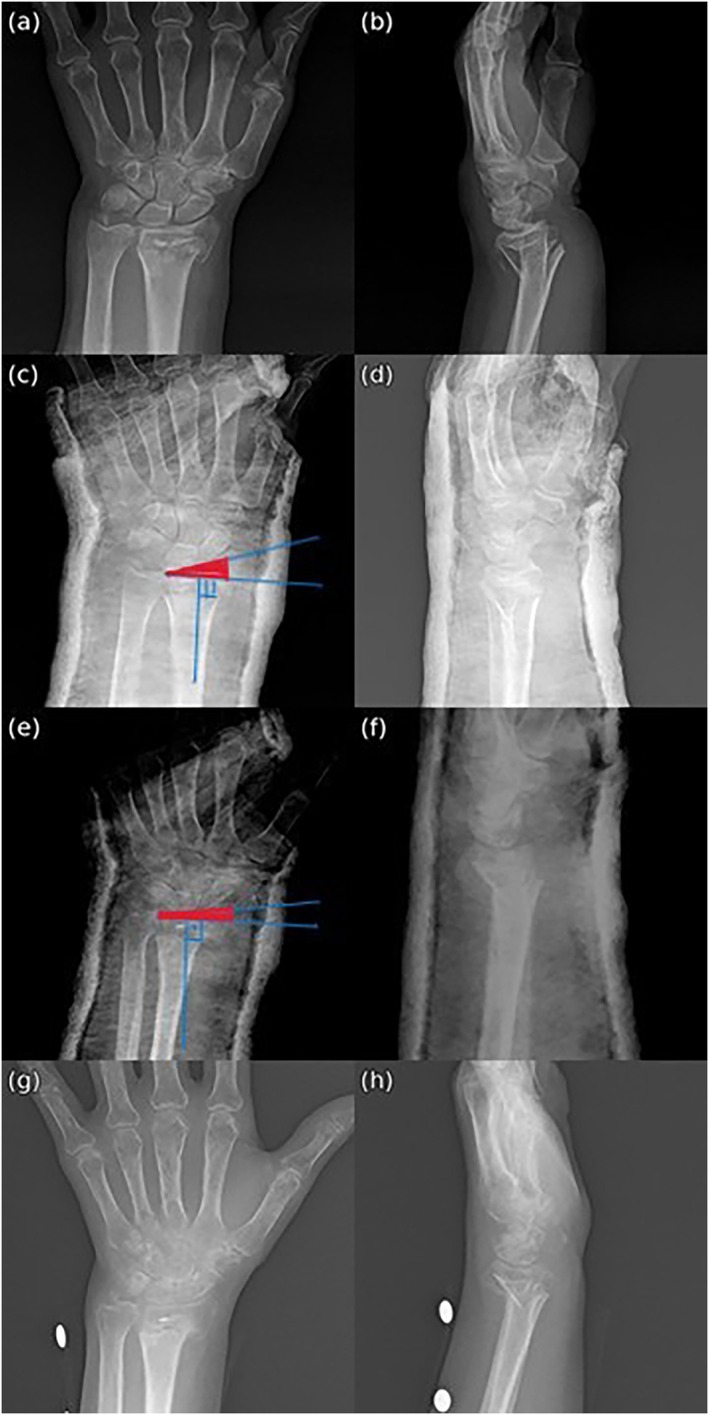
Radiographic series of a 78‐year‐old female patient with an intra‐articular distal radius fracture (AO/OTA type 23‐C2) who developed secondary loss of reduction during follow‐up. (a, b) At initial presentation, anteroposterior and lateral radiographs demonstrate the fracture pattern. (c, d) Immediately after closed reduction, radiographs obtained in a below‐elbow cast show acceptable alignment. (e, f) At 2‐week follow‐up, radiographs demonstrate secondary loss of reduction. Despite radiographic displacement, surgical conversion was not performed due to the patient's high anesthetic risk and comorbidities. (g, h) At final follow‐up (12 months), radiographs demonstrate fracture union with malunion. Radial inclination is demonstrated on selected images (c, e), showing a decrease over time.
